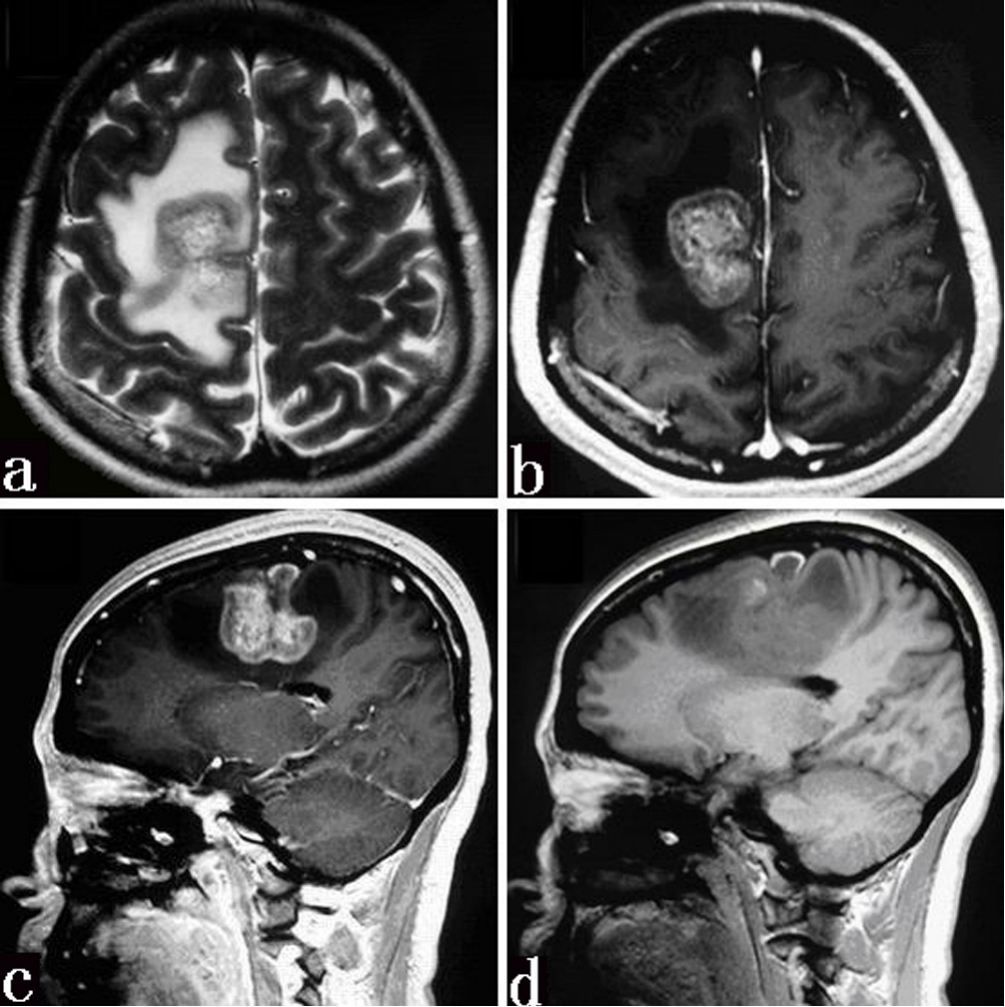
Axial T2-weighted MR image (a) showing a mass with mixed signal intensity and a surrounding edema area. On the T1-weighted image after the administration of contrast material (b and c), the mass has an inhomogeneous enhancement. Sagittal T1-weighted MR image without contrast (d) depicting a striped hemorrhage.
